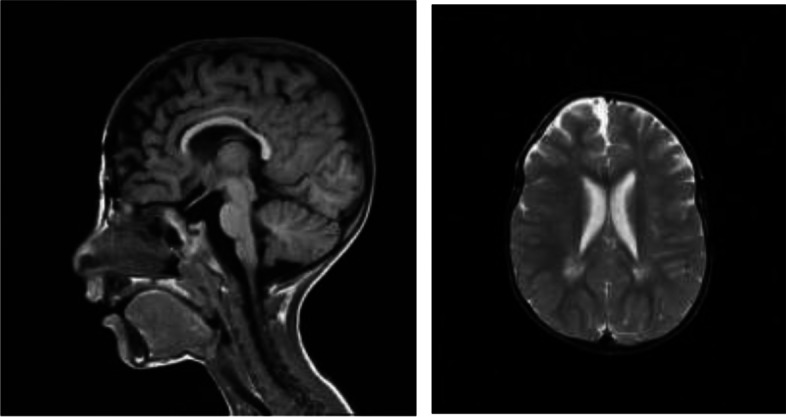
at 3 years 6 months of age, brain MRI of the patient indicated that the vascular space in the posterior horn of the ventricle was widened
